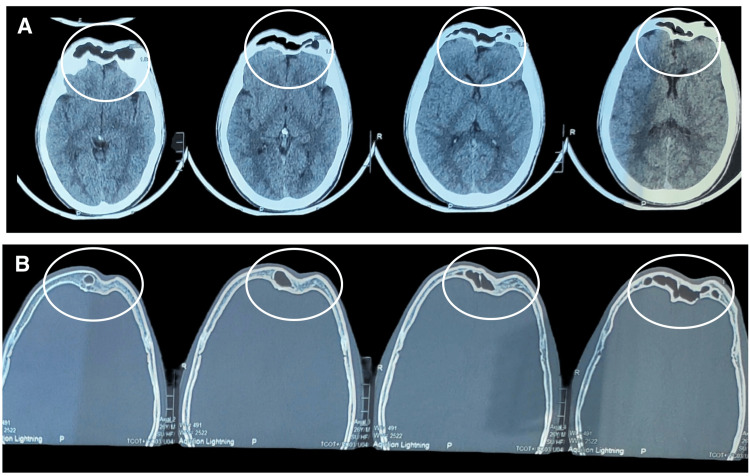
CT films depicting a fracture of the frontal bone A: soft tissue section, B: hard tissue section CT: computed tomography
